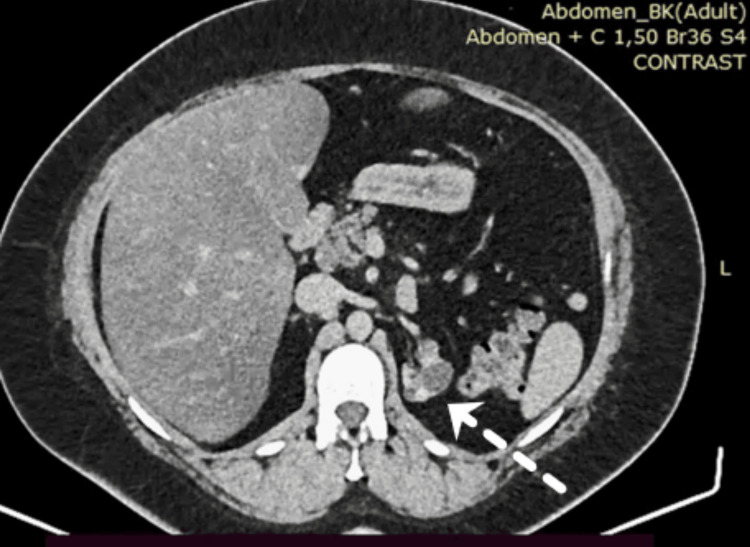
Computed tomography axial view shows a slightly enhanced mass of 1.2 × 1.1 × 0.9 cm on the left hypoplastic kidney, positioned in the renal midline (arrow).
